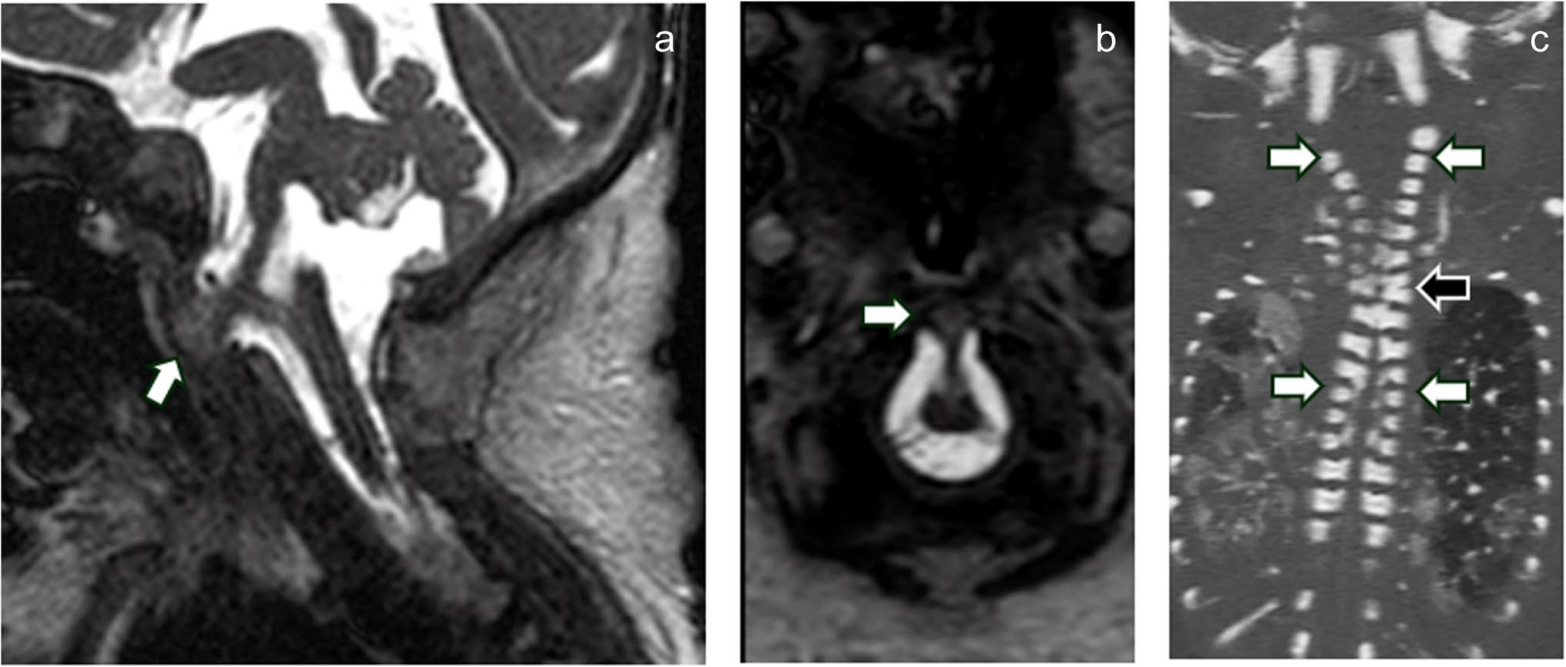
Spinal malformations. Sagittal (a) and axial (b) balanced fast-field echo T2-weighted MRI demonstrates “limited ventral myeloschisis” (*arrows* in a and b). Coronal oblique CT of the cervical and thoracic spine (c) demonstrates “zipper-like” anterior vertebral clefting (*white arrows*) and a vertebral formation and segmentation anomaly at C6-C7 (*black arrow*)
